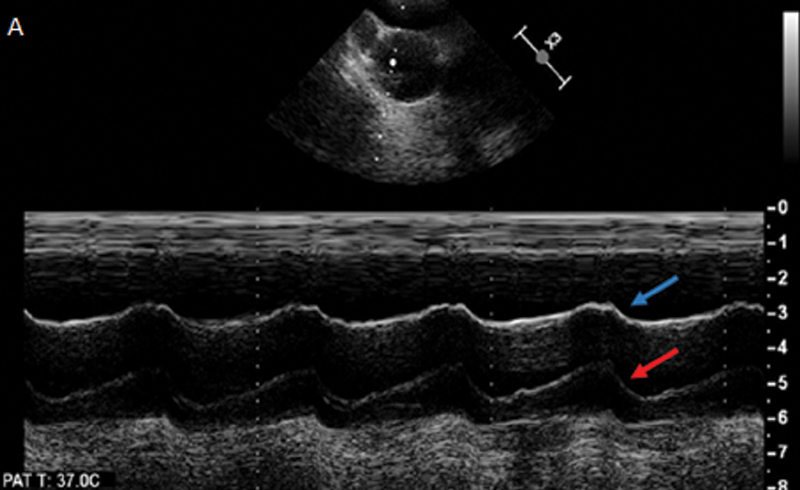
M-mode of the aorta in cross section on transesophageal echo showing reverberation artifact. The “dissection flap” (red arrow) shows perfect concordance with the aortic wall (blue arrow), suggesting artifact rather than true dissection. Image courtesy: Yale Echocardiography Laboratory.
